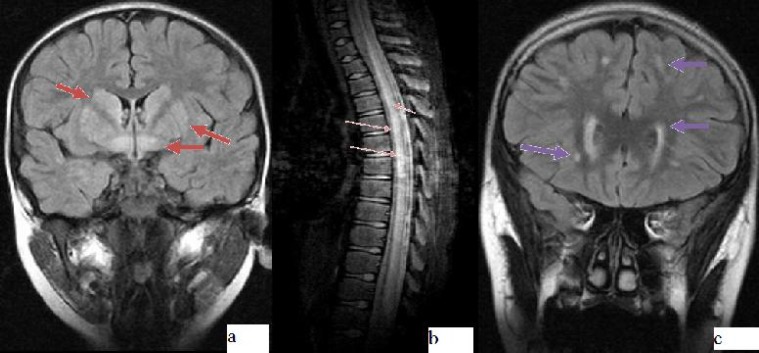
MRI findings in *M. pneumoniae* infection. a: Case 1:Hyperintensities at corpus striatum, mesencephalon, supratentorial sections on FLAIR images shown with red arrows. b: Case 2: Hyperintensities on T2 images shown with thin orange arrows. c: Case 5: Hyperintensities around lateral ventricules, frontoparietal region on T2 images shown with purple arrows.
